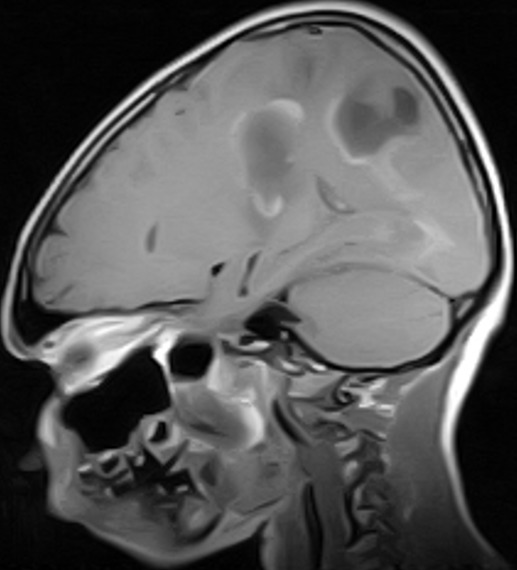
(MRI post treatment, 1yr after presentation) is a post contrast T1 w parasagittal sequence showing resolving multifocal, irregular hypointense areas with enhancing walls in the parietal region
